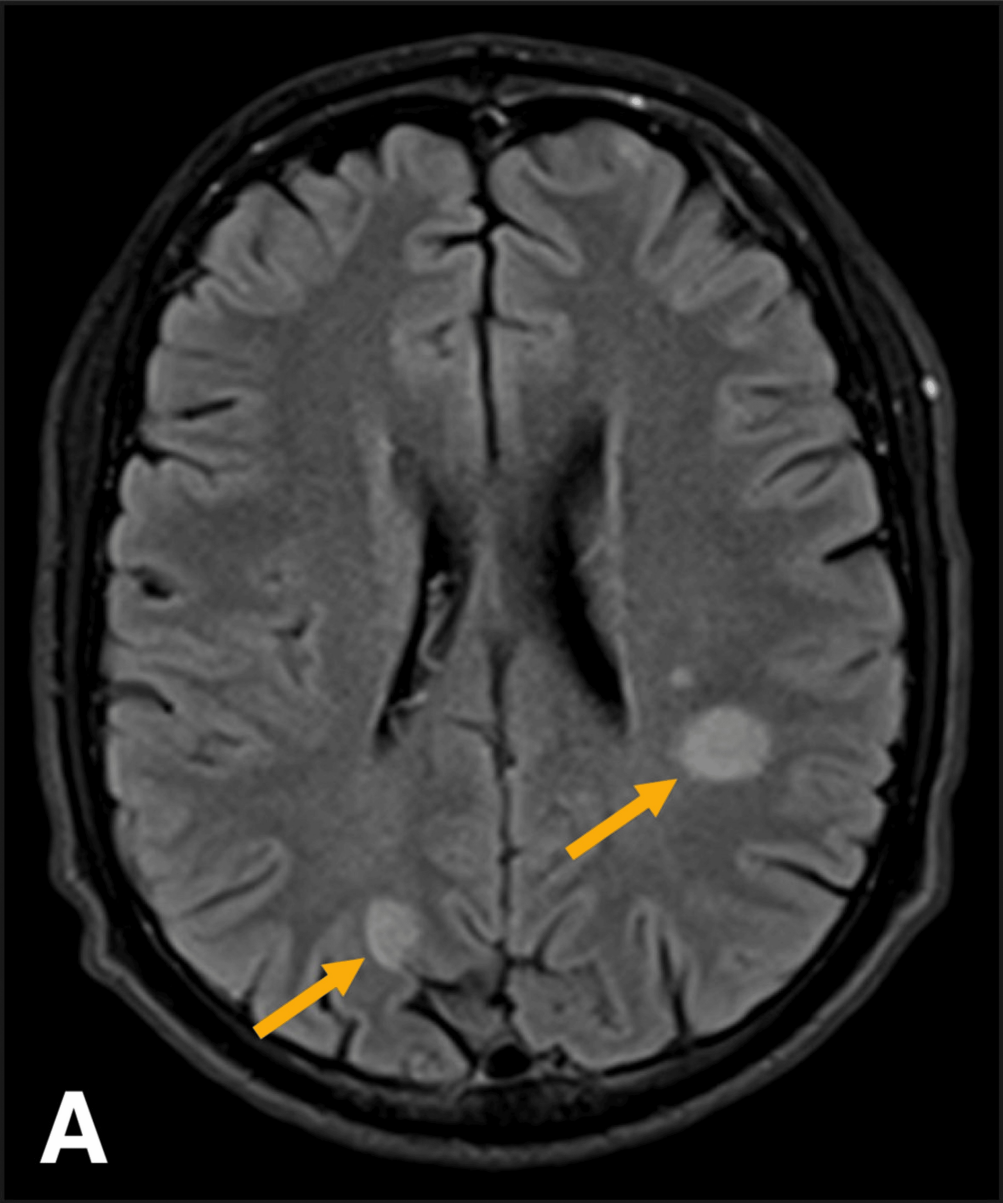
Brain MRI showing multiple focal lesions in both cerebral hemispheres
